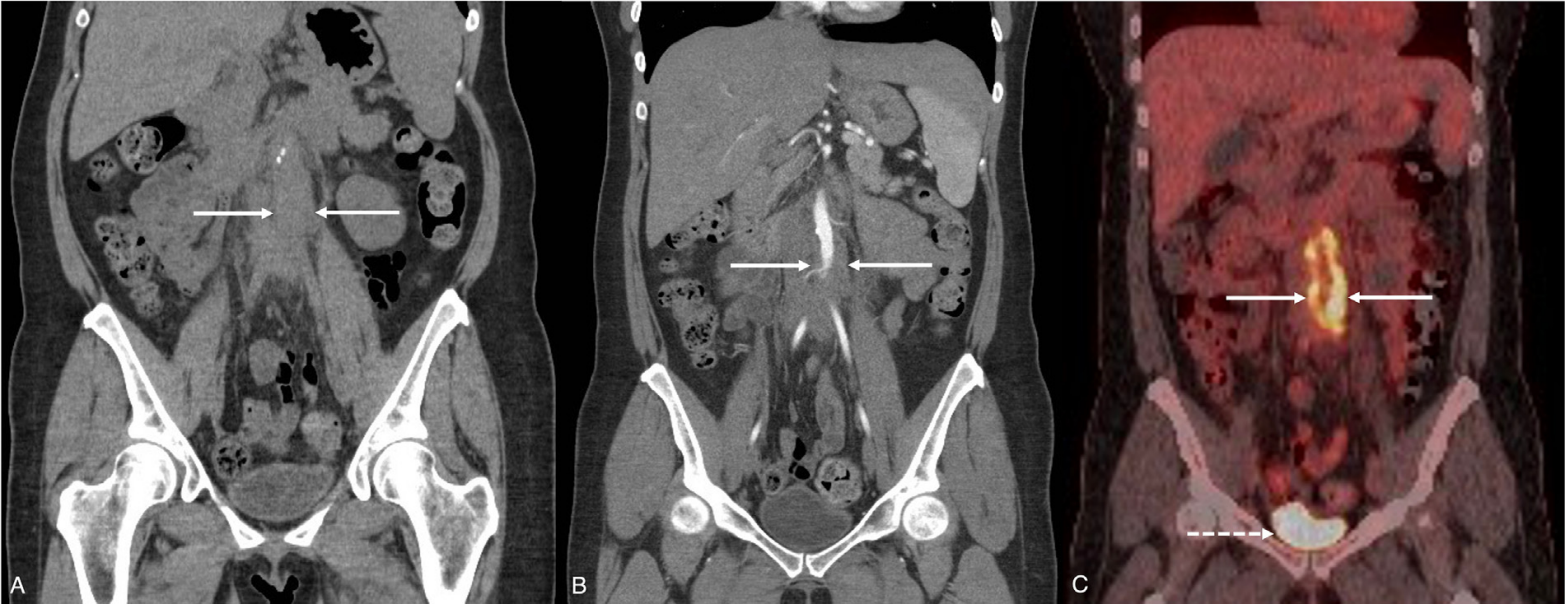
Selected coronal plane computed tomography (CT) imaging through the level of the abdominal aorta in a 42-year-old female who presented with left flank pain. (A) Initial noncontrast CT of the kidneys, ureters and bladder (patient prone) on soft tissue window (W:450, L:50; reconstructed from Fig. 1A) demonstrated subtle periaortic inflammation (between the solid white arrows). Note the foci of intramural calcification in the right infrarenal abdominal aortic wall. (B) Contrast-enhanced arterial phase imaging on soft tissue window (W:450, L:50; reconstructed from Fig. 1B) demonstrated the extent of the circumferential periaortic inflammation from the level of the infrarenal aorta to the aortic bifurcation. The opacified abdominal aorta demonstrated a narrowed caliber secondary to the periaortic inflammation (between the solid white arrows). (C) Corresponding positron emission tomography-computed tomography (PET-CT) acquisition, performed 4 days after (A) and (B), demonstrated intense fluorodeoxyglucose (FDG) activity within the circumferential wall thickening of the abdominal aorta (between the solid white arrows) and the adjacent periaortic fat stranding. Intense activity was demonstrated in the urinary bladder in keeping with expected renal excretion (dashed white arrow). No significant activity was identified elsewhere within the abdomen, pelvis, or other large vessel wall. The appearances were compatible with an isolated large vessel vasculitis or periaortic IgG4-related disease (IgG4-RD).
